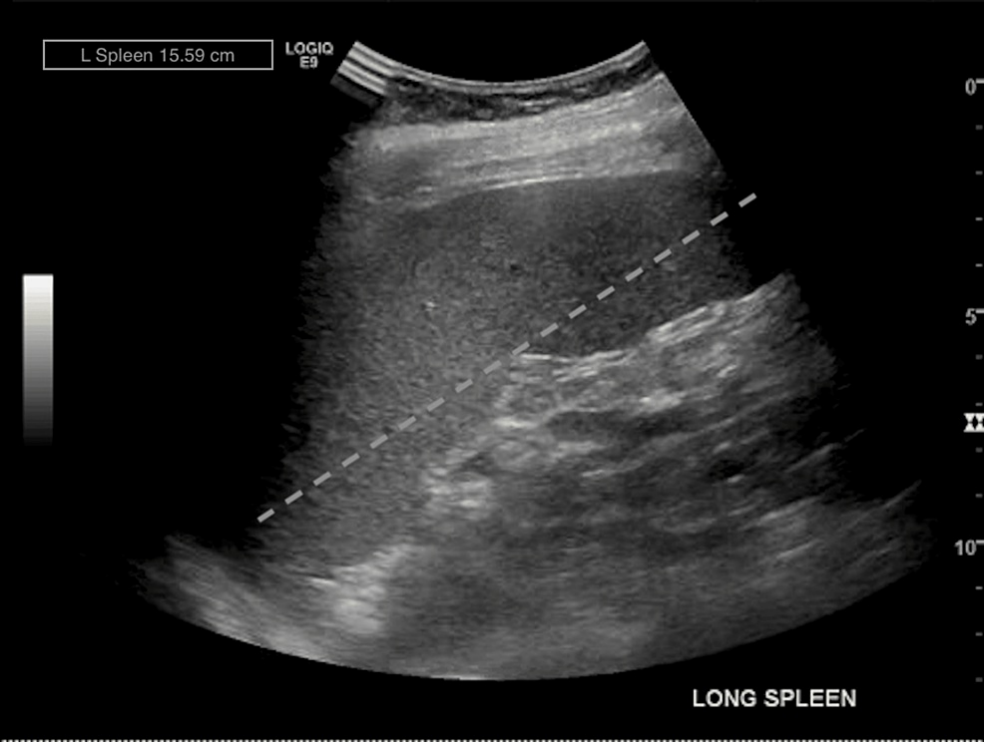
Longitudinal ultrasound of spleen
